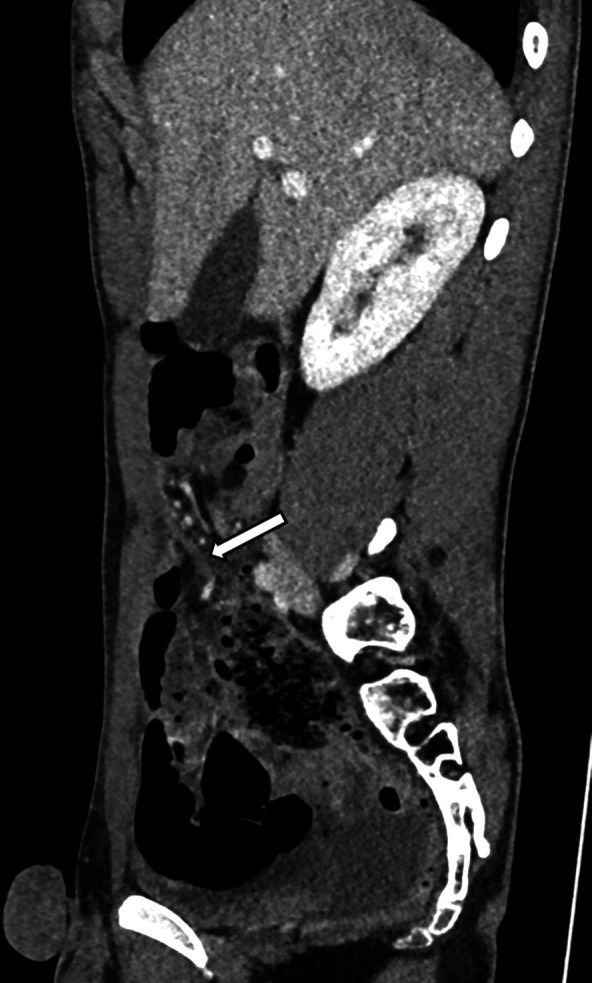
Sagittal image from a contrast-enhanced CT exam showing a fibrous band (arrow) attached to the umbilical area. This band served as the lead point around which the small bowel was wrapped.
